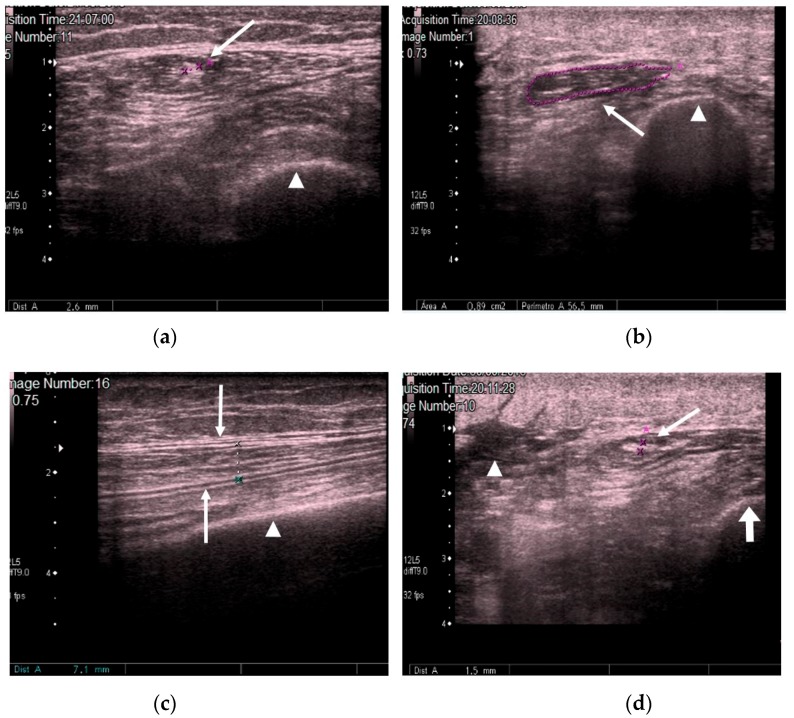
(a) Transversal QRF muscle US scan: multiorgan failure, tendon thickness of 2.6 mm (arrow), and femur (arrowhead). (b) Transversal QRF muscle US scan: multiorgan failure, cross-sectional area (CSA) of 0.89 cm^2^ (arrow), and femur (arrowhead). (c) Longitudinal QRF muscle US scan: muscle thickness of 7.1 mm (arrow) and femur (arrowhead). (d) Longitudinal QRF muscle US scan: subcutaneous edema, with intramuscular and interfacial fluid (arrowhead). QRF tendon is 1.5 mm (arrow) with the femur (thick arrow). QRF: quadriceps rectus femoris, US: ultrasound, CSA: cross-sectional area.
